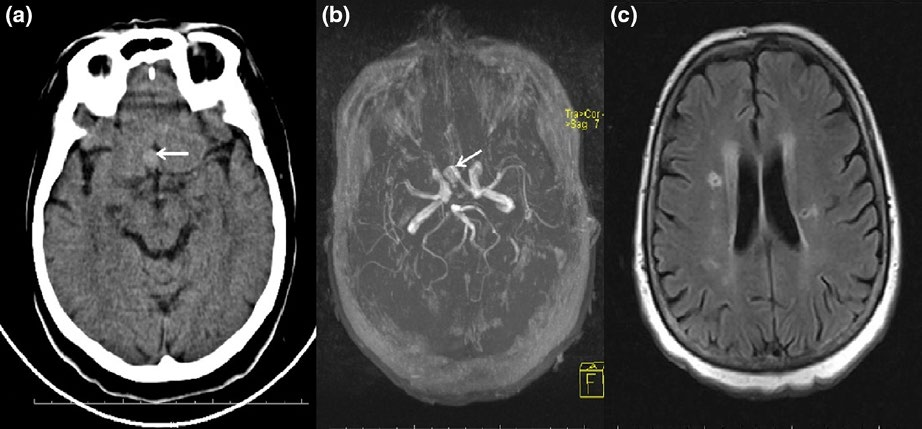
In case 6, axial cranial CT without contrast revealed a nodular hyperdense (arrow) suspicious appearance in terms of aneurysm in the anterior communicating artery location (a); the aneurysm was confirmed with 3D TOF MR angiography (b). Bilateral periventricular T2‐weighted hyperintense (c) signal changes in the deep white matter were also observed
